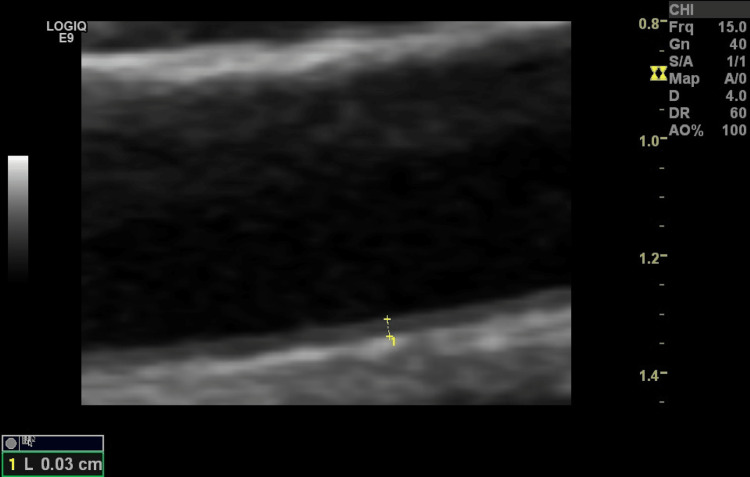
Longitudinal ultrasound image of an artery showing intima-media thickness.
